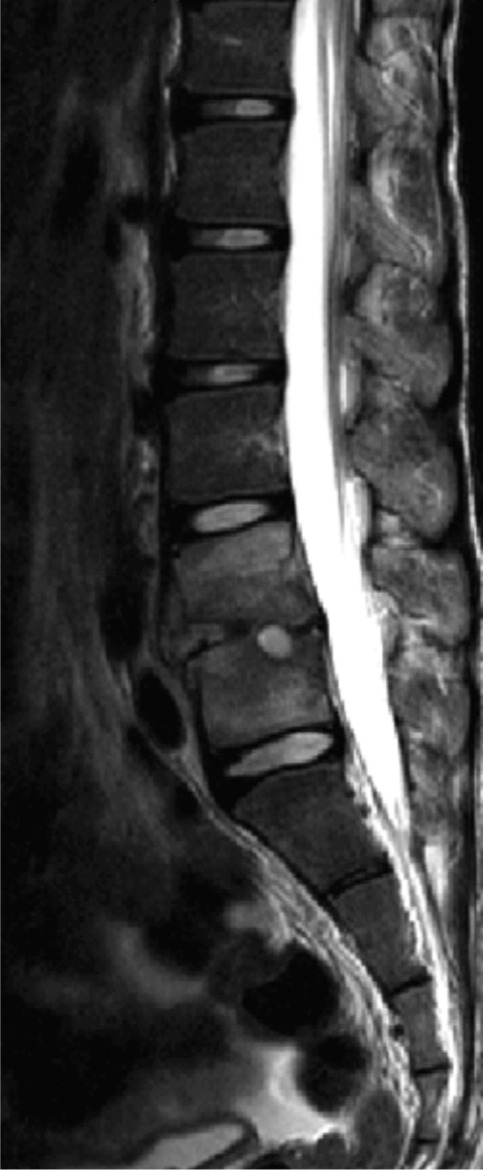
MRI mid sagittal T2 weighted image of the lumboscral spine showing L4-L5 intervertebral disc destruction.
